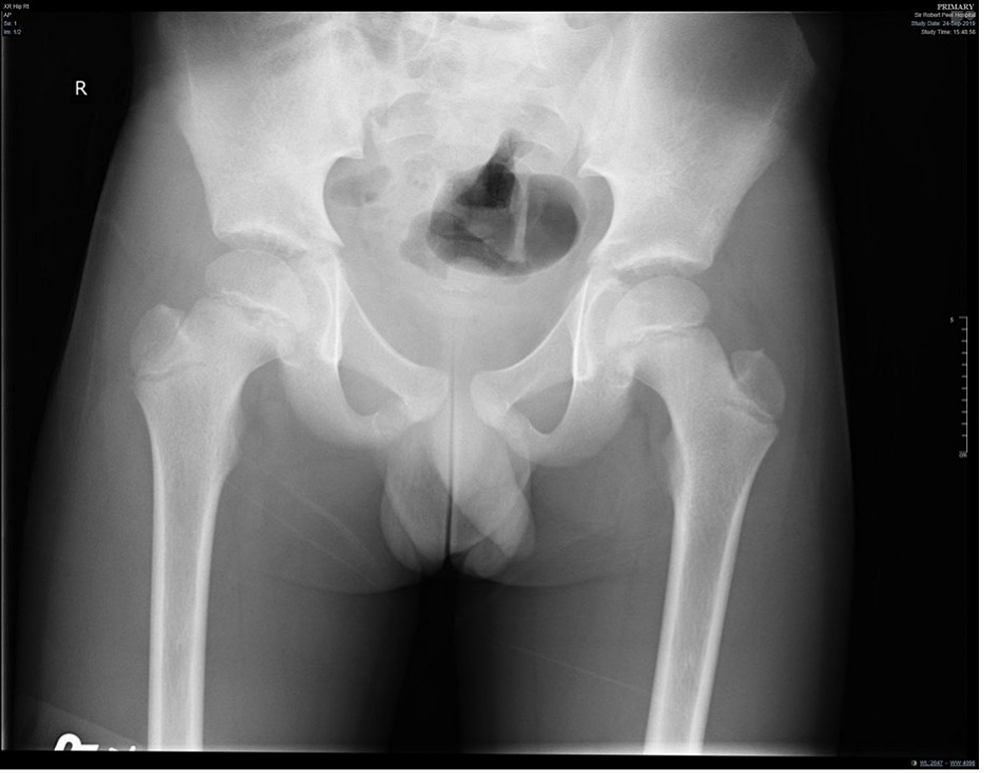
Anteroposterior pelvic radiograph showing normal pelvic anatomy, normal growth plates, and no evidence of bony injury or Perthes disease.
